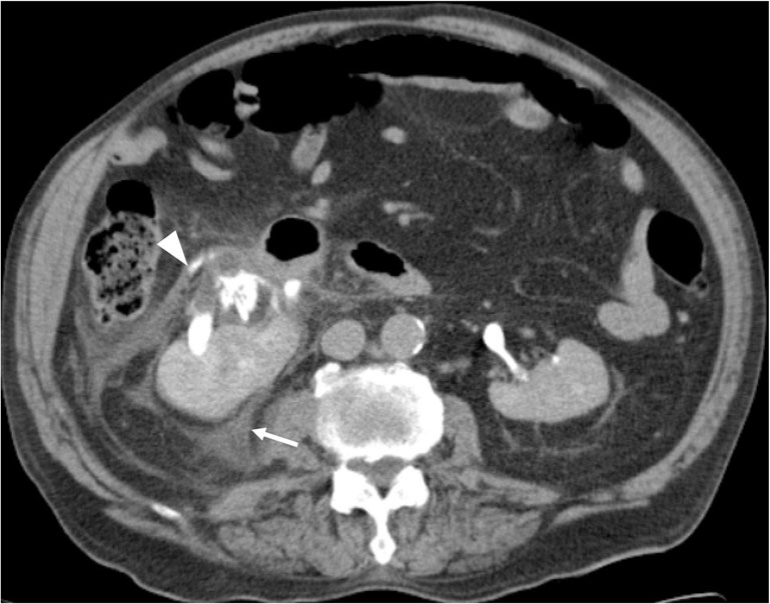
Delayed-phase CT in a patient with calyceal rupture following lithotripsy shows fluid in the perinephric space (*arrow*) and extravasation of contrast (*arrowhead*) in keeping with active urine leak
